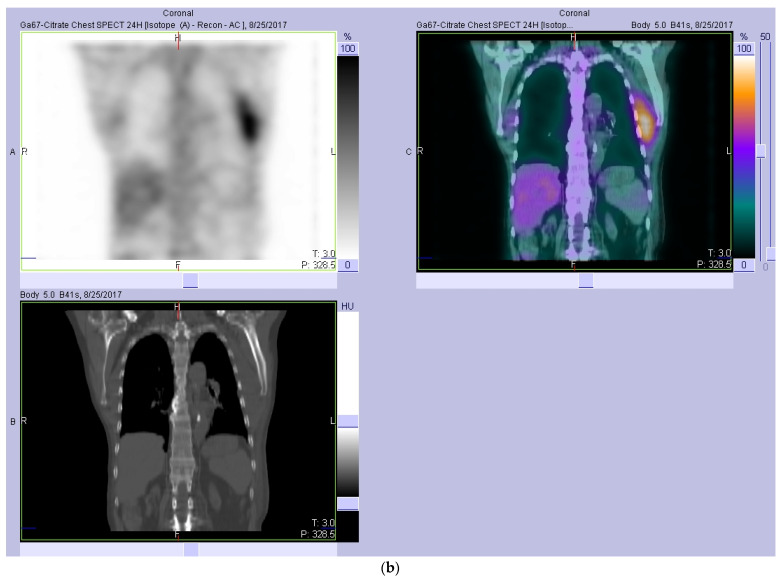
(a) CT scan of the chest. A heterogenous, relatively well-defined, soft tissue mass located along the left serratus anterior muscle, measuring approximately 5.5 × 4.2 × 2.0 cm^3^ in the superoinferior, oblique, and transverse dimensions. (b) The single photon emission computed tomography (SPECT/CT) images of a gallium scan. Note significantly greater gallium uptake at the posterolateral aspect of the left chest wall. The upper one (transverse section) and lower one (coronal section) both showed increased uptake in this mass over chest wall.
